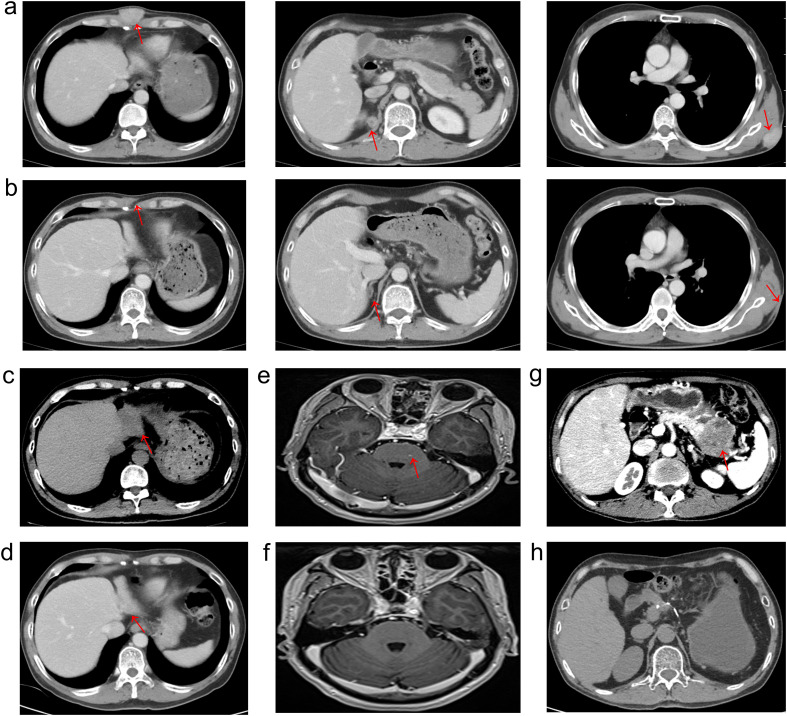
Imaging findings in the patient. (a) After the initial surgery, the lesion recurred, manifesting as subcutaneous masses located below the xiphoid process, near the edge of the right kidney, and on the left side of the back [computed tomography (CT)]. (b) Following toripalimab immunotherapy, the masses under the xiphoid process, adjacent to the right kidney, and in the back had decreased in size (CT). (c) After combined treatment with toripalimab and anlotinib, follow-up CT in September 2020 revealed a soft-tissue mass in the hepatogastric space. (d) Upon discontinuation of anlotinib and continuation of toripalimab, follow-up CT demonstrated regression of the soft-tissue mass in the hepatogastric space. (e) Abnormal hyperintense foci and an enhancing lesion in the left pons, suggestive of metastasis [cranial magnetic resonance imaging (MRI)]. (f) After chemotherapy and immunotherapy, the pontine enhancing focus was resolved (MRI). (g) A soft-tissue mass in the pancreatic tail, suggestive of pancreatic metastasis (positron emission tomography-CT). (h) The latest follow-up imaging indicated stable disease. Red arrows indicate the tumor location.
